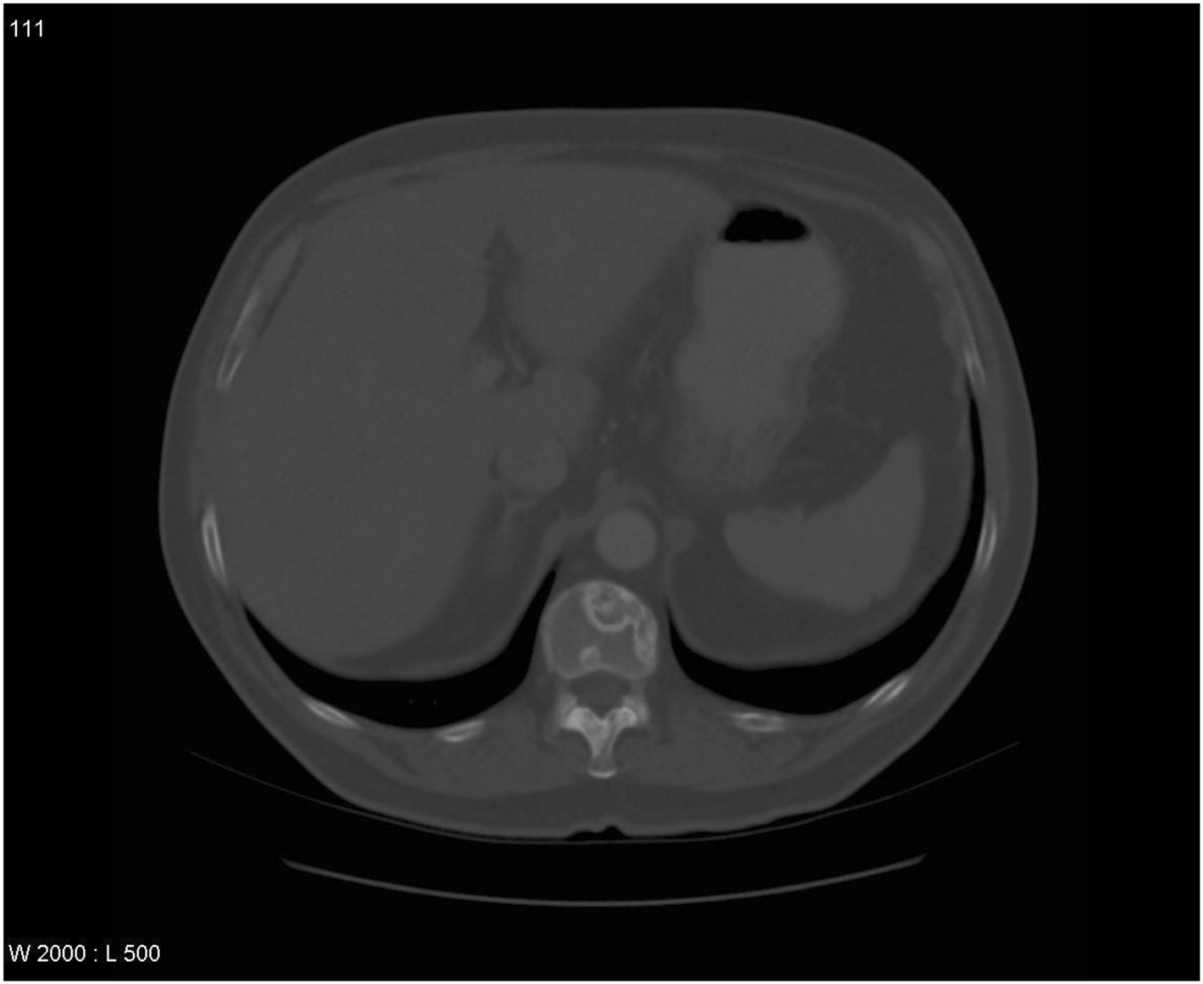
Computed tomography scan in a patient with adenocarcinoma of the prostate, multiple bone metastases and uncomplicated bone pain (bone lesions not causing neurological complaints and without a high risk of pathological fracture), no extra-osseous extension: treated with 8 Gy x1.
